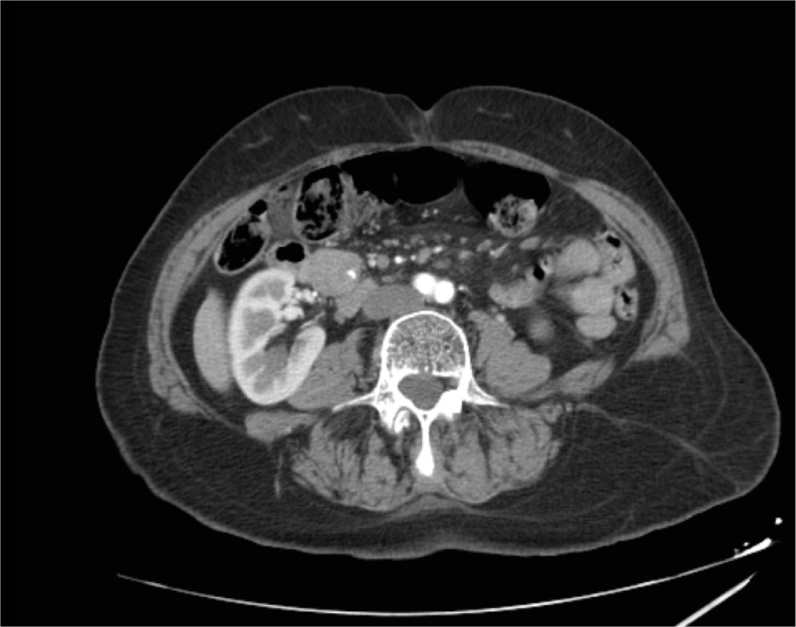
Arterial phase abdominal CT – tumor mass in the pancreatic uncinate process with inner calcification of 3.4/2 cm.
